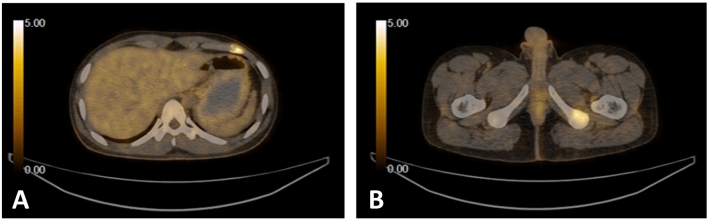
PET-CT demonstrating FDG avidity (A) over the left 6th rib and (B) left ischium.
